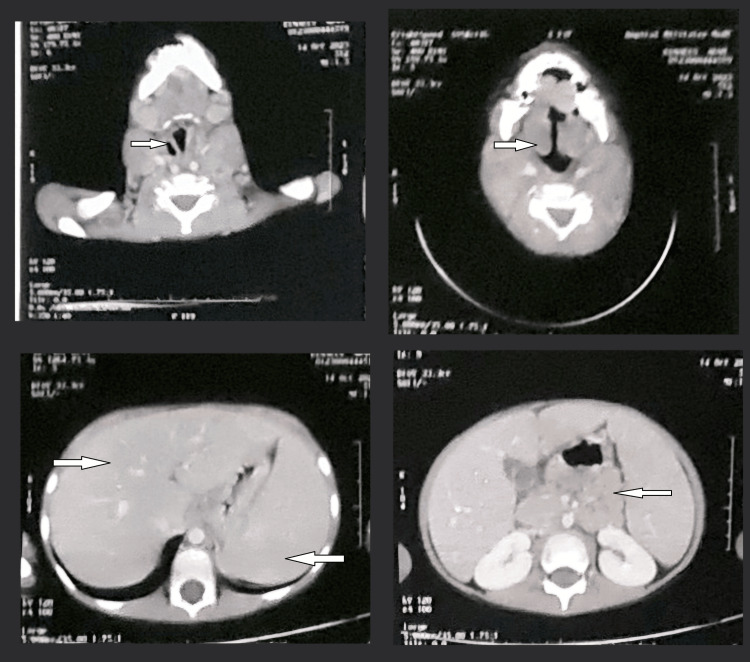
Images from the cervical and thoraco-abdomino-pelvic CT scan of the second patient showing thickening of the nasopharynx, reducing the airway with bilateral cervical adenopathies and homogeneous hepatosplenomegaly with supra and subdiaphragmatic adenopathies.
